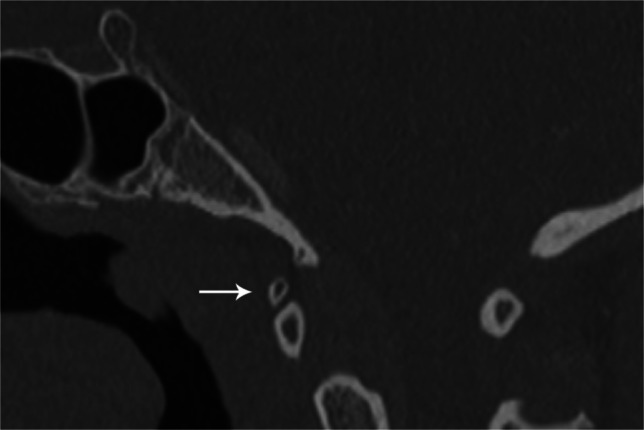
Sagittal CT of the skull base in an 11-year-old female. Accessory ossicle (*arrow*) anterior to the anterior arch of C1. Possibly a remnant of the hypochordal bow. Accessory ossicles can occur in various locations about the craniocervical junction
